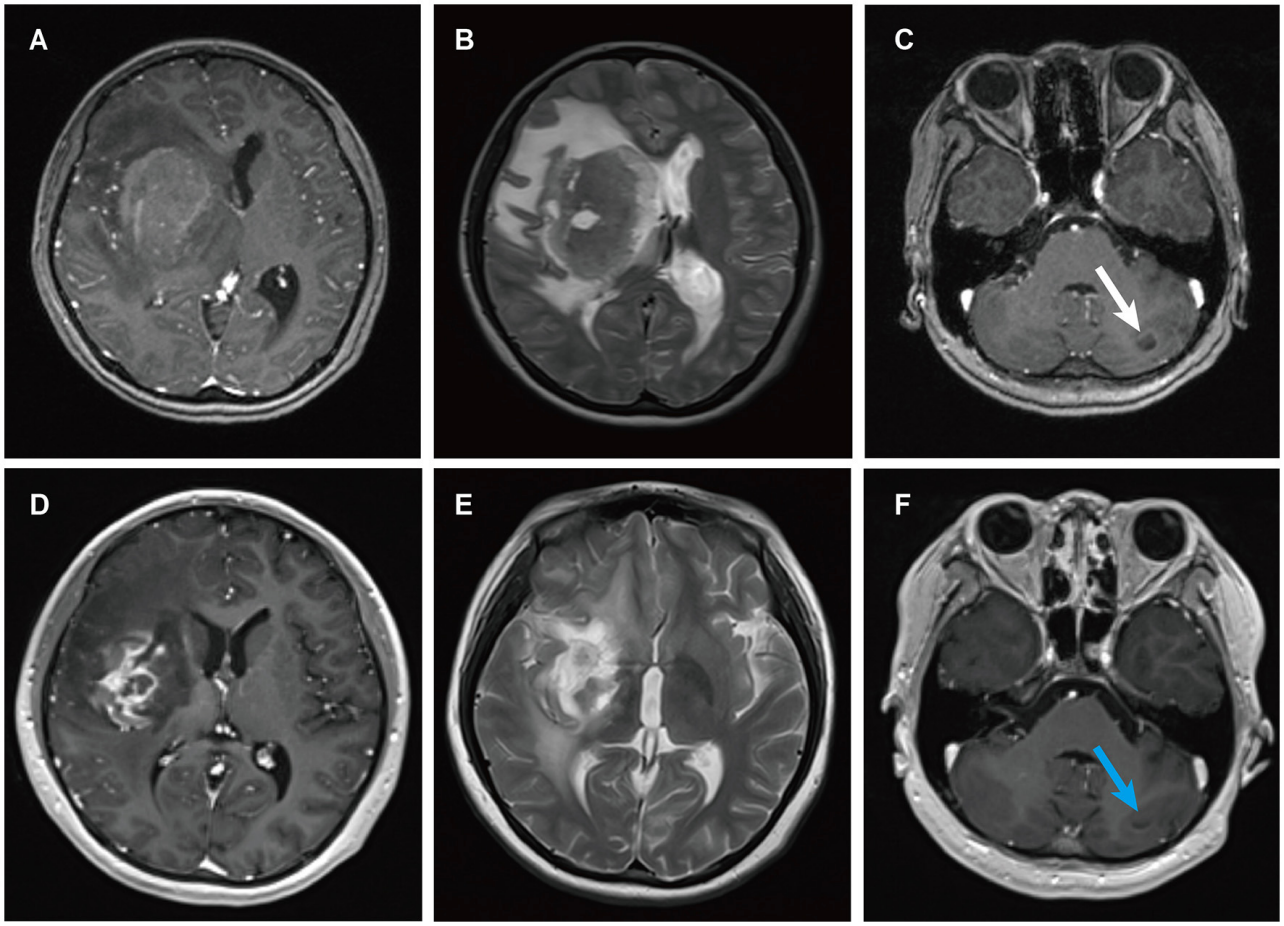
MRI findings of patient 2. Preoperative enhanced MRI prior to brain biopsy showed a ring-enhancing lesion in the right basal ganglia area on T1-weighted images (A), with extensive edema surrounding the lesion in the right basal ganglia area on T2-weighted images (B), and abnormal signal intensity in the left cerebellum on T1-weighted images [(C) white arrows]. Enhanced MRI scans obtained four months after the diagnosis of PCNS PTLD, persistent ring enhancement lesion in the right basal ganglia on T1-weighted images (D). Continued perilesional edema in the right basal ganglia on T2-weighted images (E). Additionally, the abnormal signal intensity in the left cerebellum persisted on T1-weighted images [(F) blue arrows].
